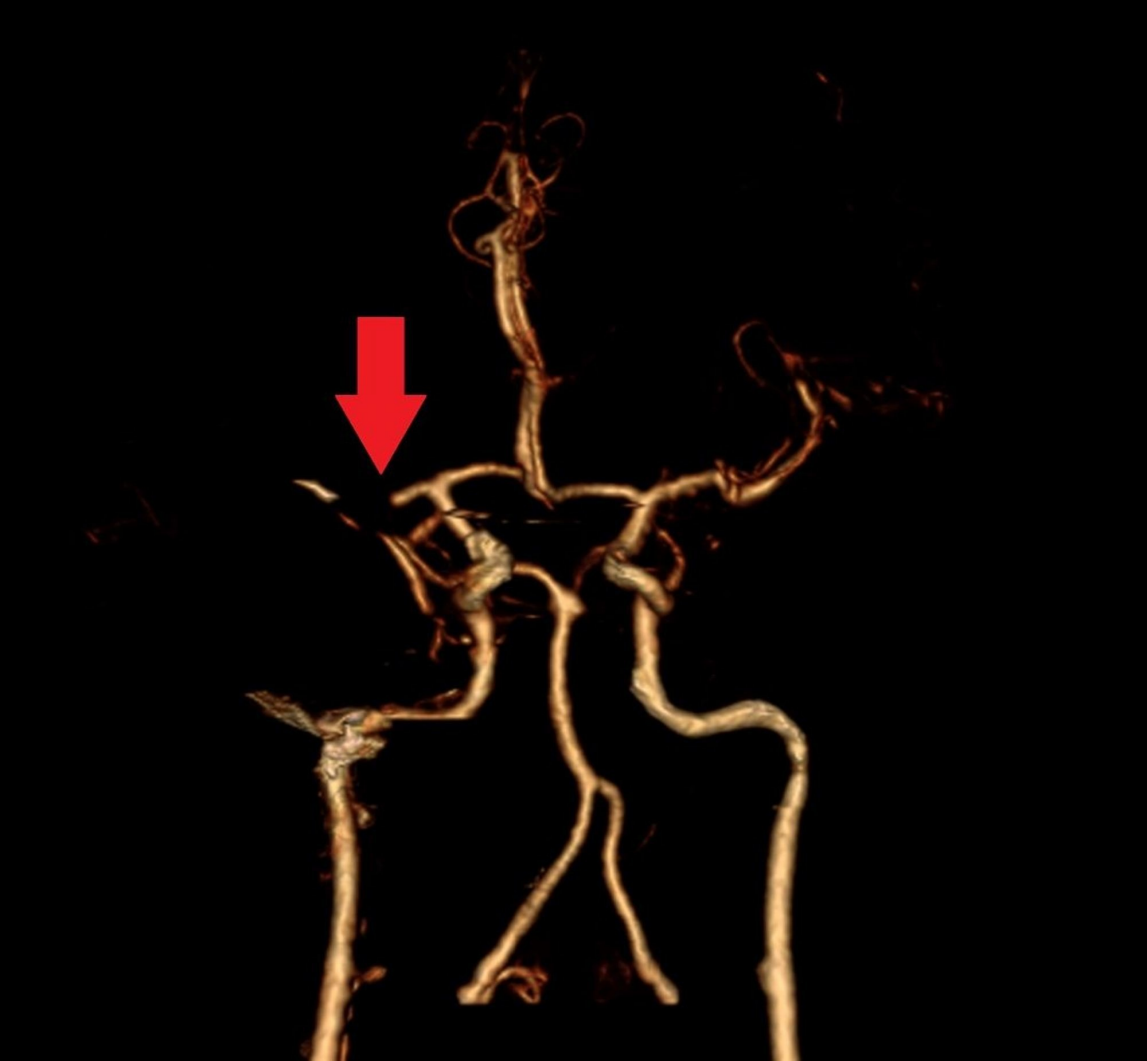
CT angiogram head showing occlusion of the right middle cerebral artery Complete occlusion of the M1 segment of the right middle cerebral artery is seen on CT angiogram (red arrow) CT: computed tomography
